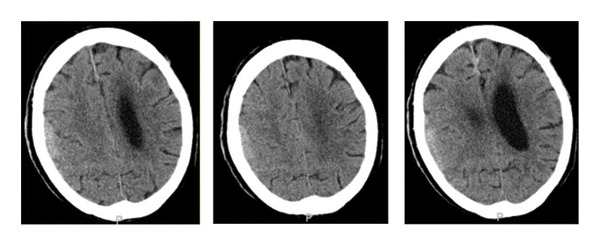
Axial noncontrast CT head images at the level of the lateral ventricles and centrum semiovale. CT demonstrates subdural hematomas along the right cerebral convexity and anterior falx cerebri, chronic infarct involving the left basal ganglia, chronic ischemic change/gliosis in the left frontal periventricular white matter, and ex vacuo dilatation of the frontal horn.
